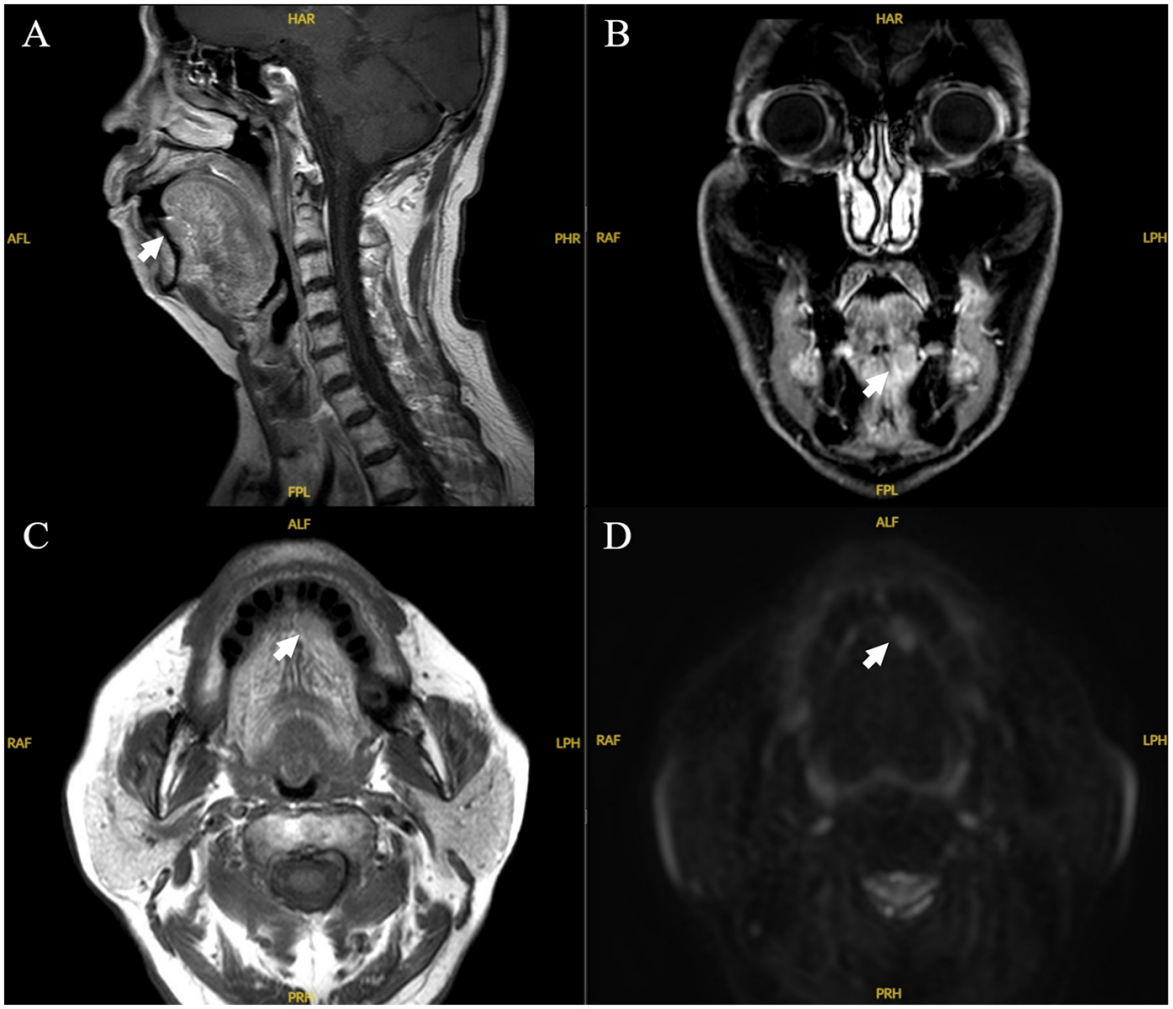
MRI imaging of the case. Sagittal (A), coronal (B), axial (C), and axial diffusion weighted imaging (D) MRI images. There was a 2.0 cm × 1.8 cm hyperintense unicystic, regular mass with clear margins, located under left tongue.
